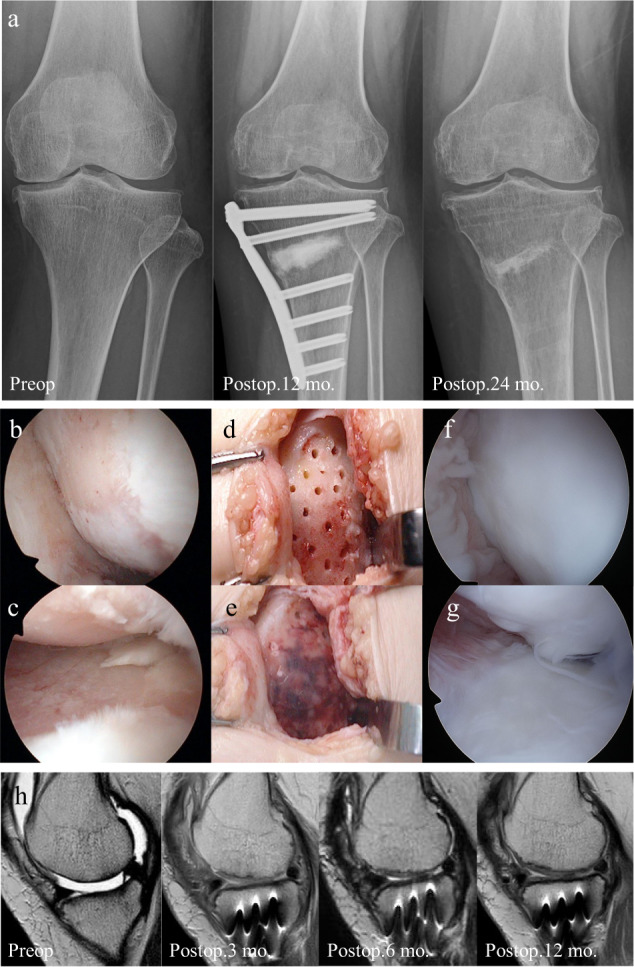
Case presentation of regenerative therapy (patient 5). X-ray photographs (a), arthroscopic images (b–g), and magnetic resonance imaging (h). a X-ray preoperative photograph of the left knee confirmed a varus deformity and narrowing of the medial joint space. X-ray photograph 12 months postoperatively showed that the alignment was maintained after OWHTO. At 24 months postoperatively (12 months after implants were removed), the alignment was maintained and beta-tricarcium phosphate graft has been absorbed. b, c Preoperative cartilage defect of the medial femoral condyle and kissing lesion of tibial plateau caused exposure of the subchondral bone. d Intraoperative pictures of medial femoral condyle after microfracture. e PD sheets were transplanted just as putting on it without suturing. f, g The presence of regenerated cartilage was confirmed at the second look arthroscopic view (12 months postoperatively). h Sagittal view of the medial femorotibial joint. T2-weighted images were obtained using a 3.0-Tesla MRI. Time course from the preoperative cartilage defect to regenerated cartilage after surgery. The preoperative MR image shows a broad area of the cartilage defect in the medial condyle of the femur, a kissing lesion in the tibia, and the collection of synovial fluid. The regenerated cartilage was detected 3 months after surgery and was maintained with time. The irregular subchondral bone caused by marrow stimulation was initially detected and became smooth by 12 months.
